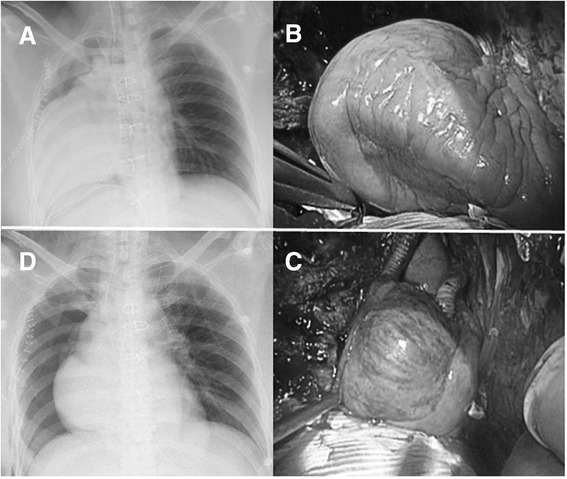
a Chest X-ray showing protrusion of the heart into the right thorax. b Intraoperative view showing cardiac herniation from the repaired pericardial defects. c Intraoperative view showing the heart returned to its normal position. d Chest X-ray showing that the heart was returned to its normal position after re-thoracotomy
